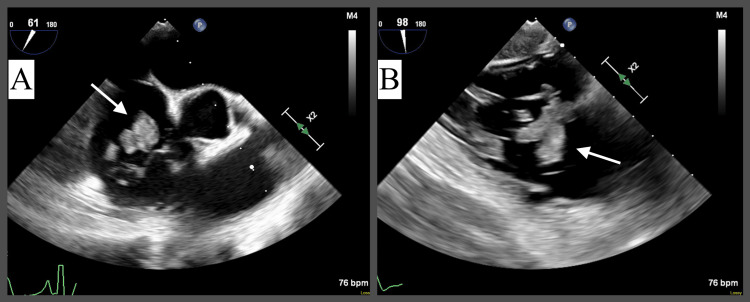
Preoperative transesophageal echocardiogram (TEE) with evidence of large, irregularly shaped, highly mobile vegetation (indicated by the white arrows) on the tricuspid valve leaflet measuring approximately 4 cm x 2 cm. A: Mid-esophageal view at 61° focusing on tricuspid valve vegetation. B: Transgastric view at 98° focusing on tricuspid valve vegetation.
